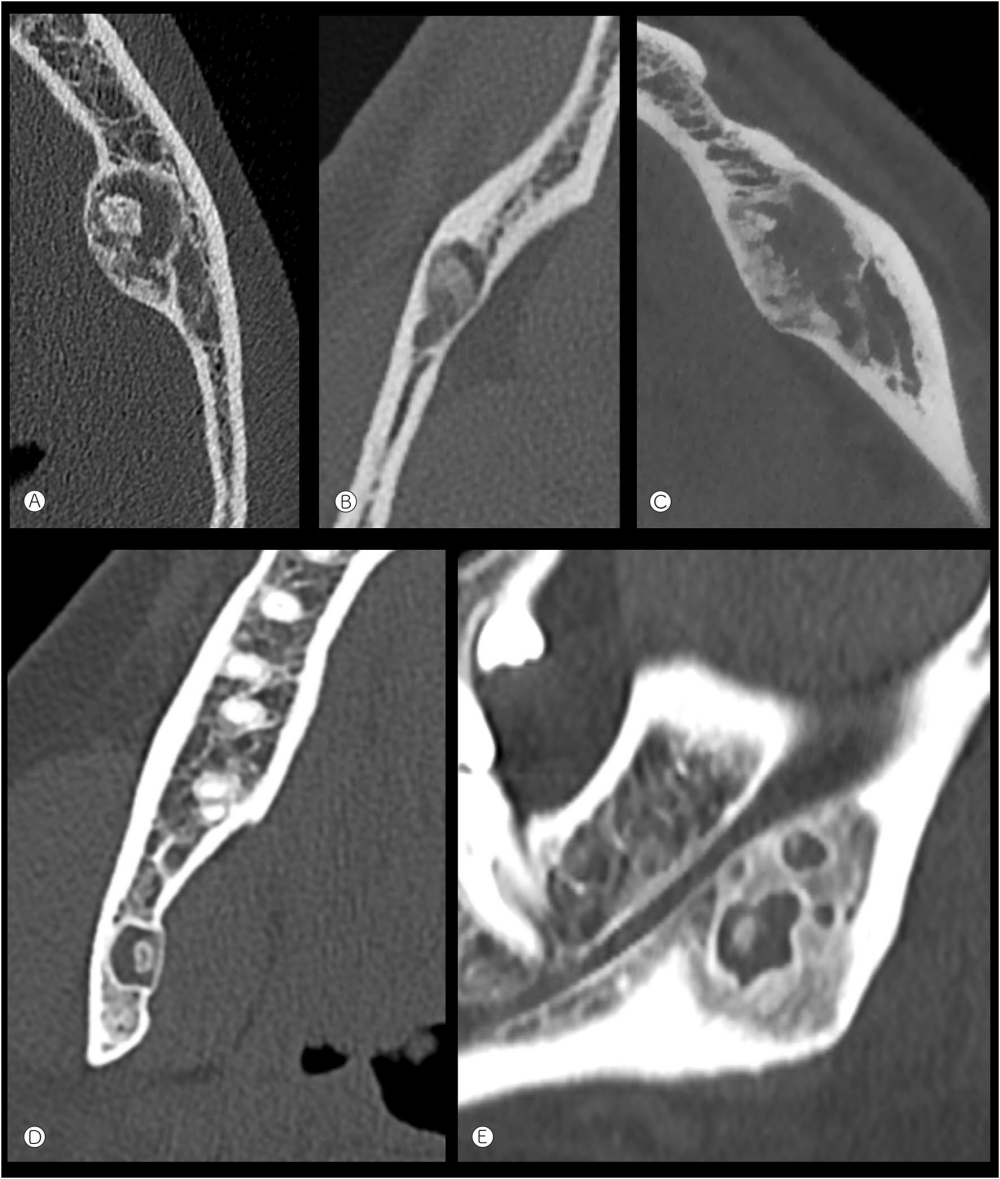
Mandibular lesions. A Intracortical tumor in the body of the left mandible with a thin sclerotic margin and amorphous central calcification (case 16, axial CT). B Intramedullary tumor in the body of the right mandible showing a thin sclerotic border, endosteal scalloping and focal ground glass density centrally (case 17, axial CT). C Intramedullary expansile tumor in the left mandible with a peripheral margin of sclerosis (similar pattern to several of the frontal lesions, case 15) (axial CT). D and E Axial CT (D) and sagittal reconstruction (E) showing a tumor with atypical features at the angle of the right mandible. Multilocular lucencies with thin sclerotic margins, central mineralization, cortical thinning and expansile remodeling of the medial cortex adjacent to the larger lesion. There is adjacent medullary sclerosis (case 19)
